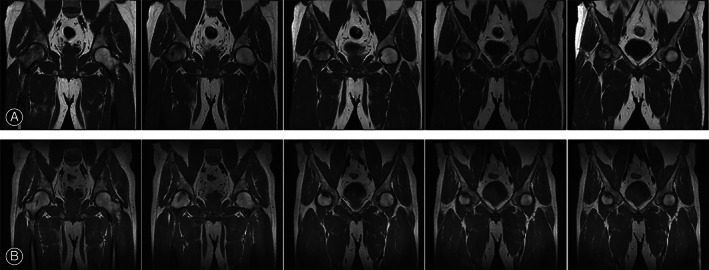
MRI of osteonecrosis of the right hip demonstrates that bone marrow edema obviously disappeared; femoral head remained spherical and no progress in necrotic area of femoral head 5 year later. (A) MRI of osteonecrosis of the right hip pre‐operation. (B) MRI of osteonecrosis of the right hip 5 years post‐operation.
